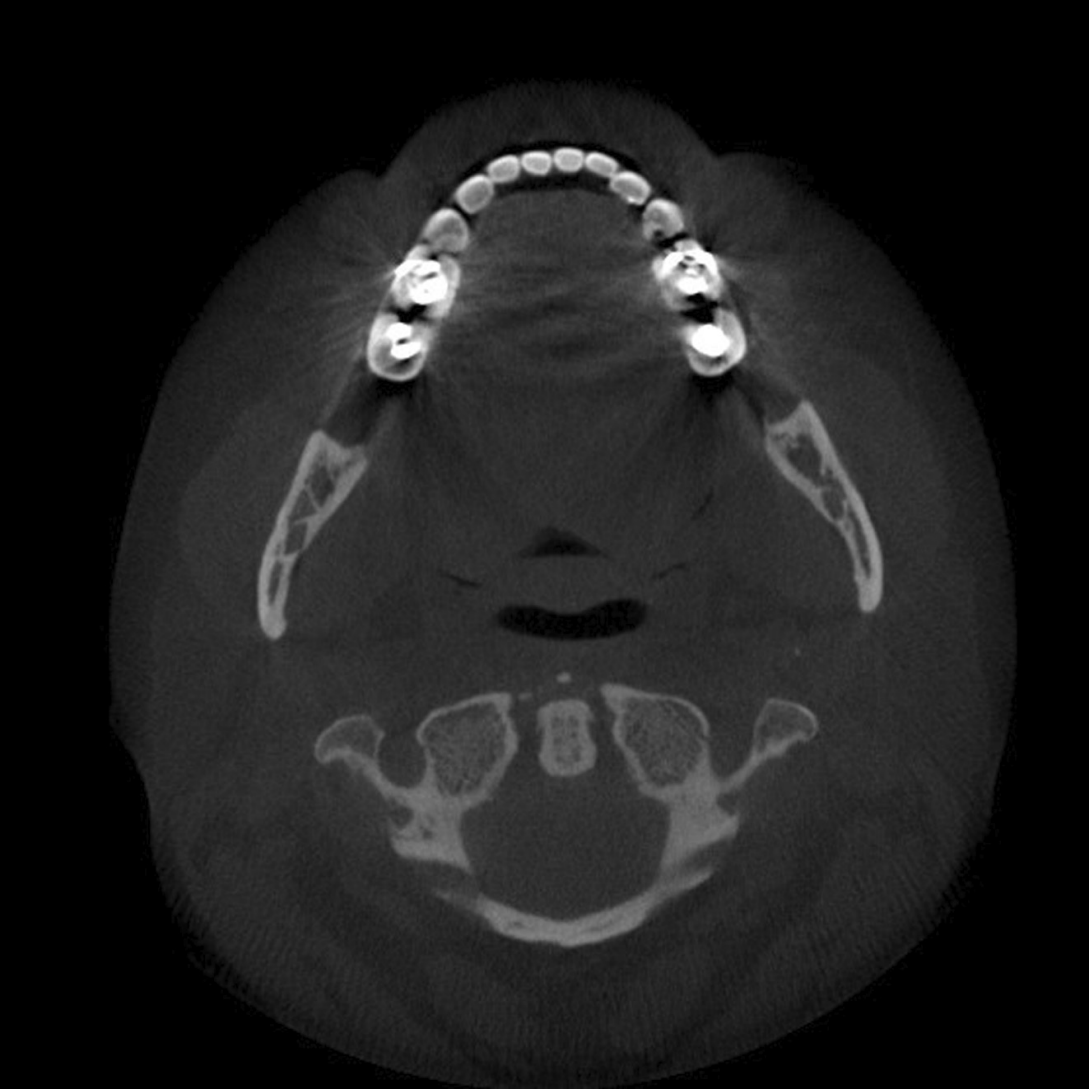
Cone beam CT scan showed edentulous maxilla, thin cortical contours, sclerotic cancellous bone surrounding the teeth and bone loss of mandibular teeth
